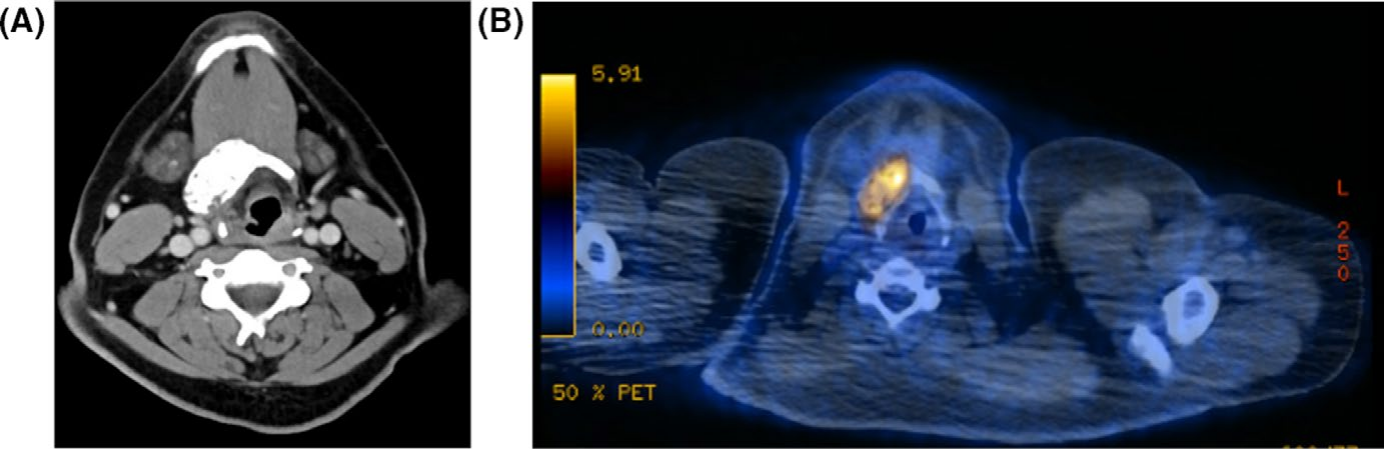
Axial imaging demonstrating a hyoid mass. A, Axial computed tomography (CT) image at the level of the hyoid bone demonstrating an expansile bony lesion within the hyoid measuring 4.1 × 2.3 × 2.8 cm. B, Positron emission tomography (PET)/CT image showing an FDG‐avid mass located within the hyoid bone measuring 4.3 × 2.0, which corresponds to the expansile mass seen on CT scan
